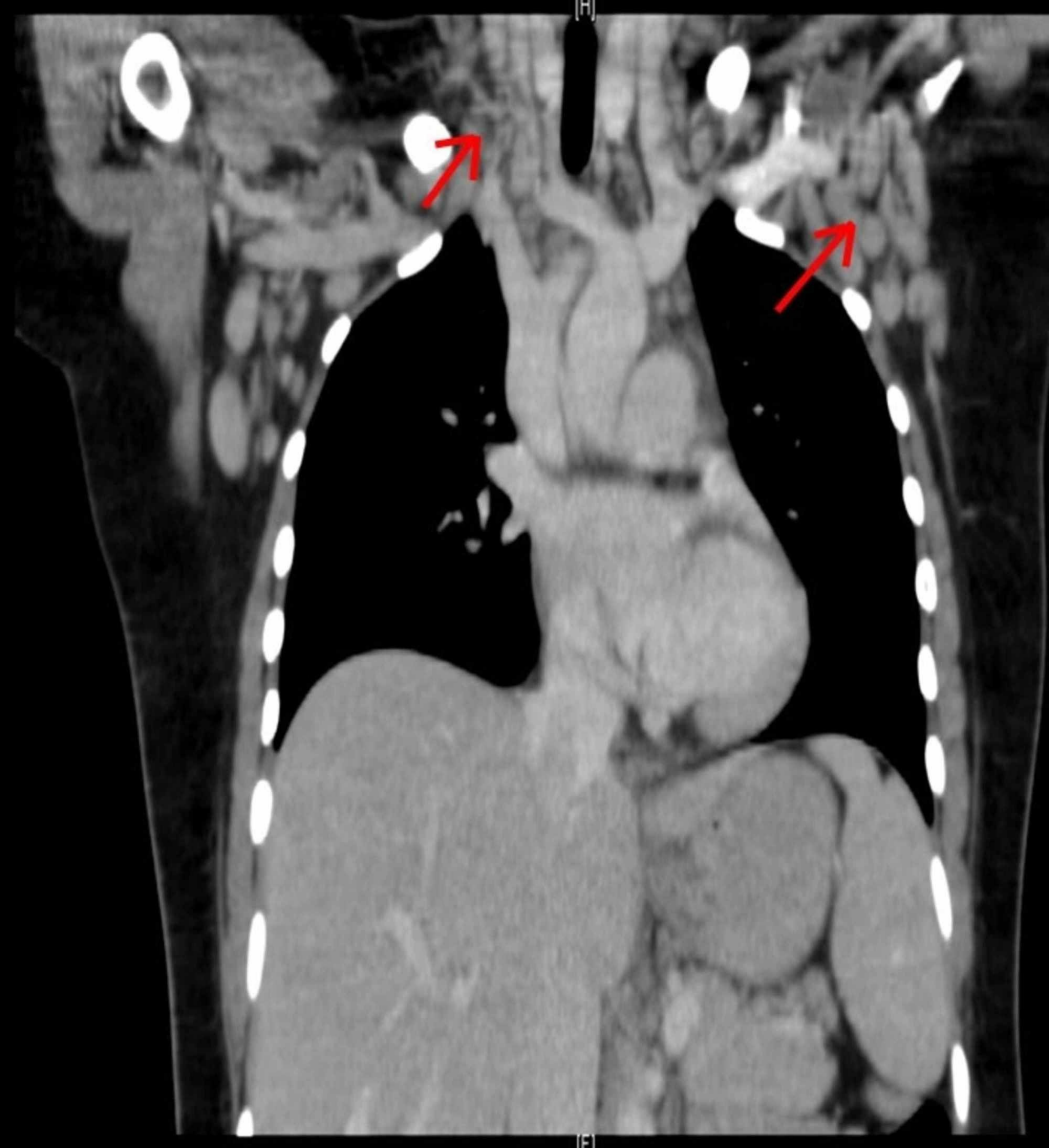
Coronal CT scan of the chest showed pathologically enlarged cervical, supraclavicular, axillary and mediastinal lymph nodes.
